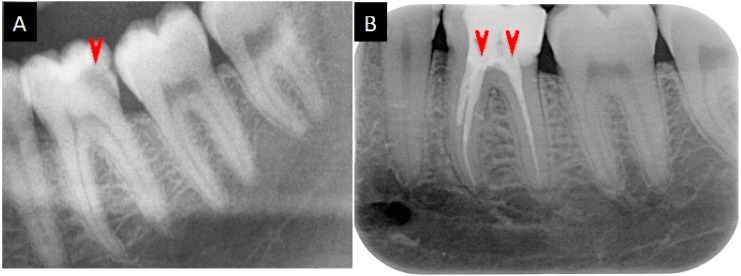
Molar 36 of a 25-year-old male patient before and after treatment. (A) The radiolucent area in the X-ray image indicates decay. (B) The sealed root canals after treatment with GS. The red arrowheads mark the decayed area and the sealed root canals, respectively.
